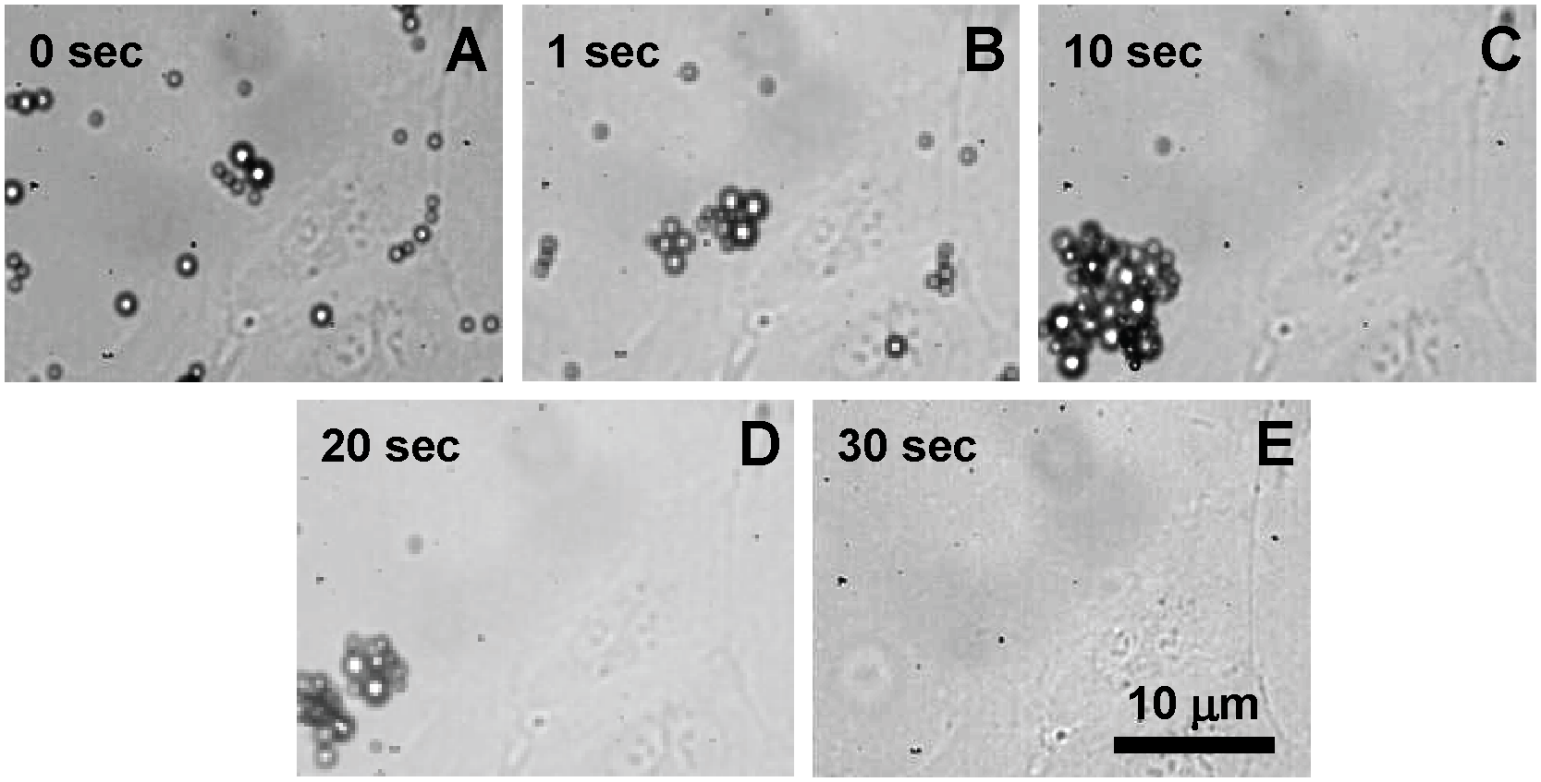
Optical recording of tMB on HUVECs during ultrasound treatment. (A) before treatment. (B-E) tMB displacement, clustering, and destroyment during 30 s insonification (1MHz, 80 kPa PNP, 10,000 cycles, repetition rate of 20 Hz, 30 s insonification treatment, SPIO added at -5 min with respect to the start of insonification).
